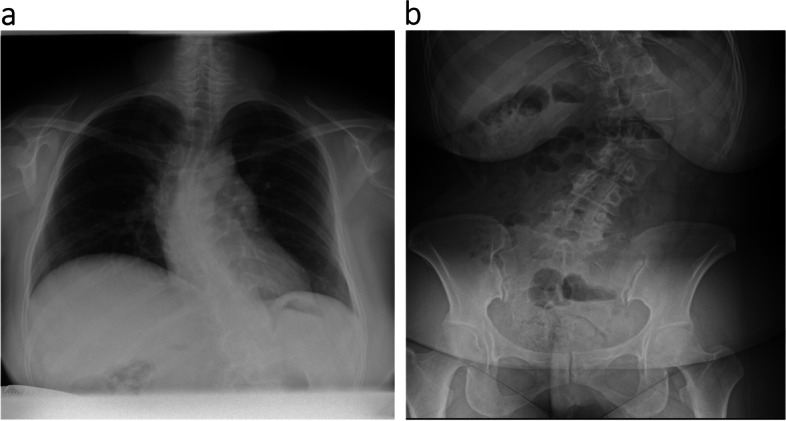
X-ray exams for a pregnant woman with moderate scoliosis. a Chest X-ray before delivery revealed that her thoracic Cobb angle was 43°, and her upper lumbar spine was confirmed to have scoliotic change. b Abdominal X-ray after delivery revealed that her lumbar Cobb angle was 41° and rotated
